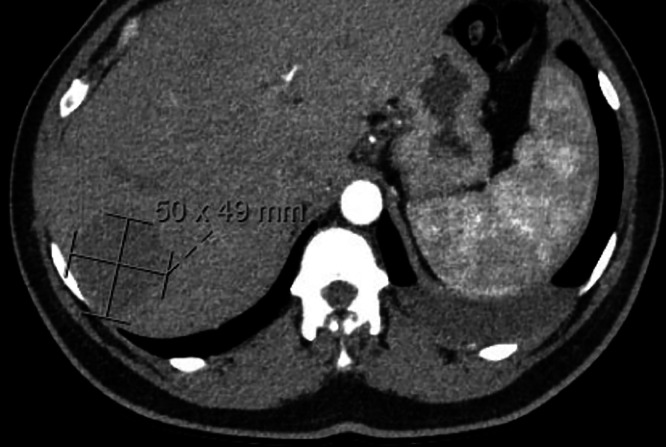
Abdominal CT demonstrating a 5 cm hypodense lesion in the right liver lobe with peripheral nodular enhancement in the early arterial phase, consistent with a hepatic hemangioma. This incidental finding was confirmed based on imaging characteristics and required no further intervention.
